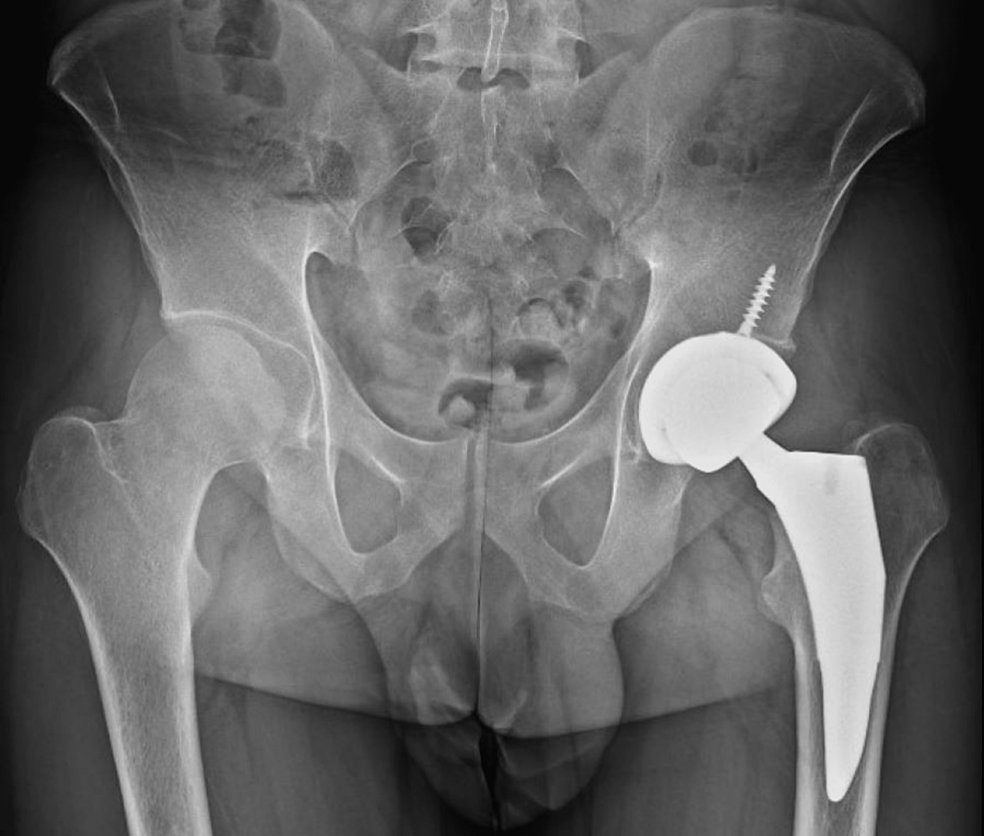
AP plain film one month post-operative revealing prosthesis in good position
